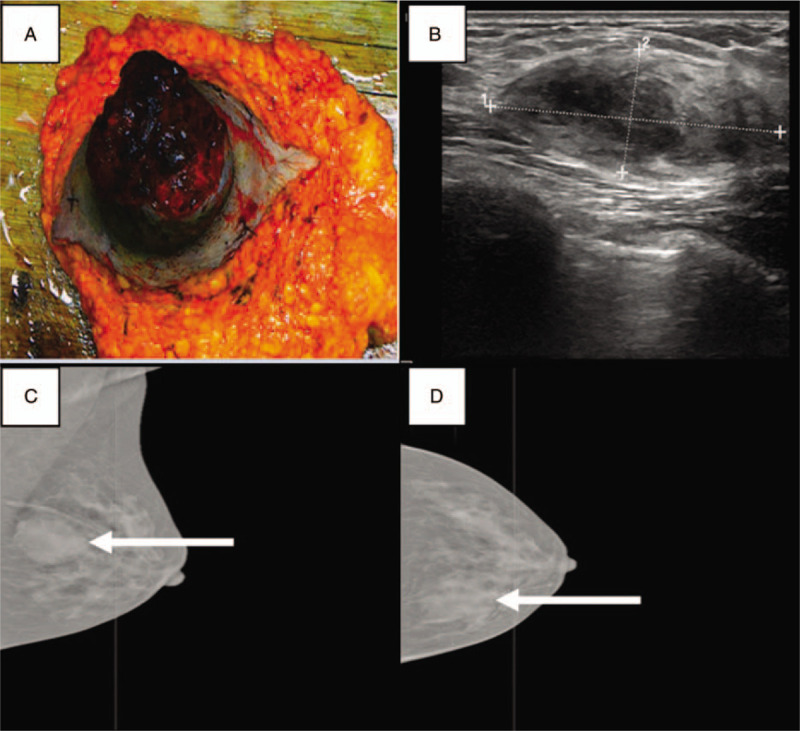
A, Diffuse large B-cell lymphoma in the left breast with multiple necrotic and hemorrhagic foci. B, The ultrasonography result showed that low echo nodule in the left breast. C and D, Molybdenum palladium roentgenograph of left breast revealed an irregular soft tissue mass (arrow).
